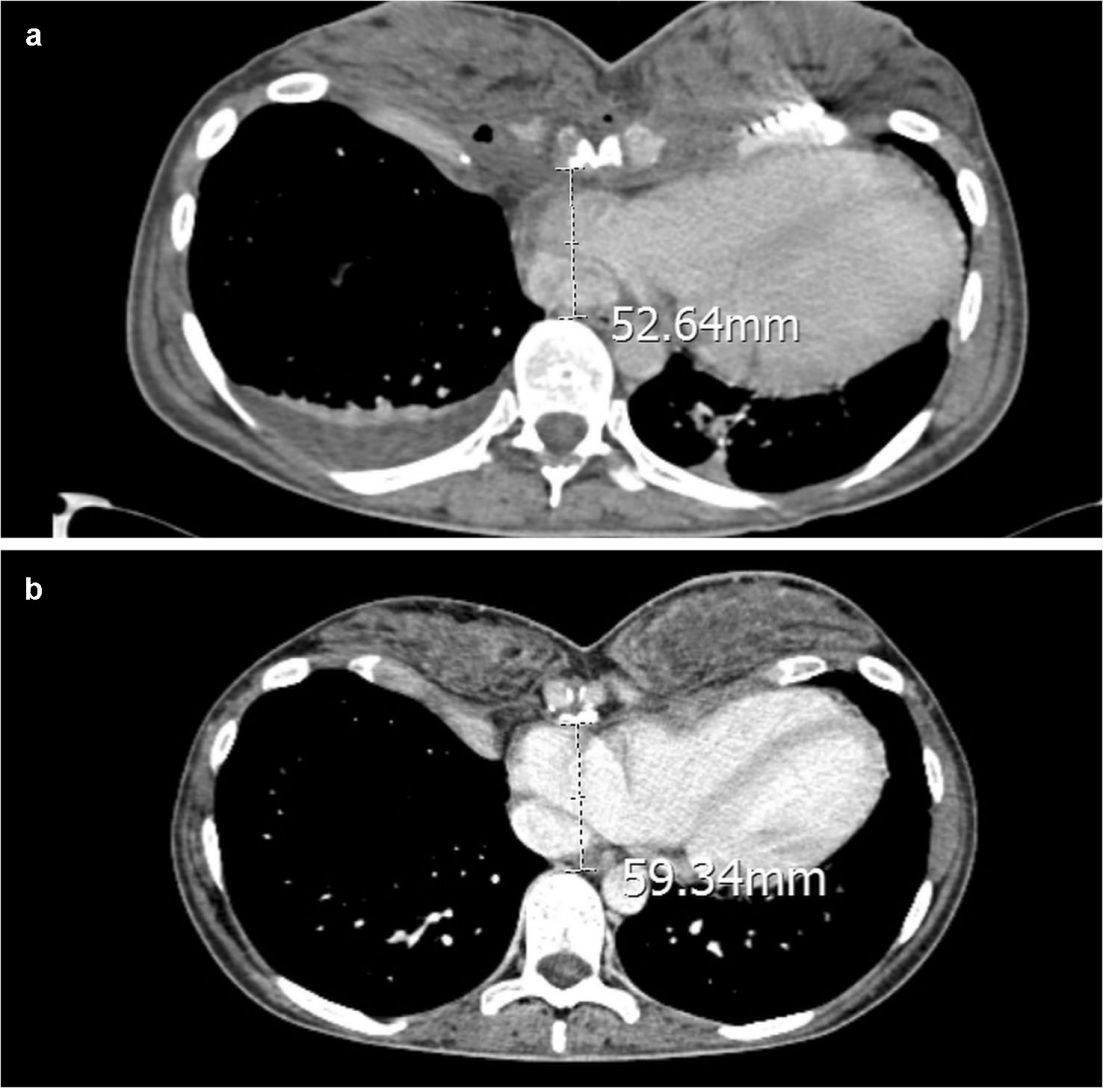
a Postoperative axial CT scan at 3rd day showing improvement of antero-posterior diameter. b Postoperative axial CT scan at 6 months showing further improvement of antero-posterior diameter
